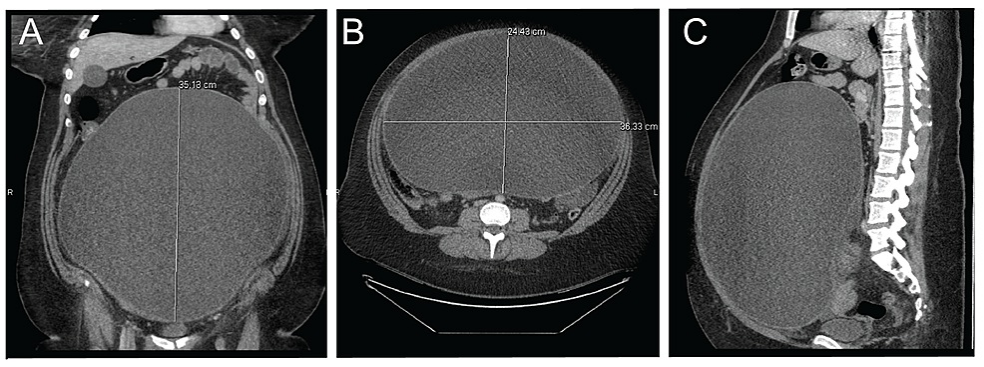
Computed Tomography Reveals an Abdominal Mass Multiplanar CT image of the abdomen and pelvis with IV contrast showing a large cystic mass extending to the pelvis and the upper abdomen which displaces bowel loops. The mass measures approximately 35 x 24 x 36 cm with a thin, peripheral rind measuring 5mm and is suspicious of a neoplasm. A: Coronal plane. B: Axial plane. C: Sagittal plane.
